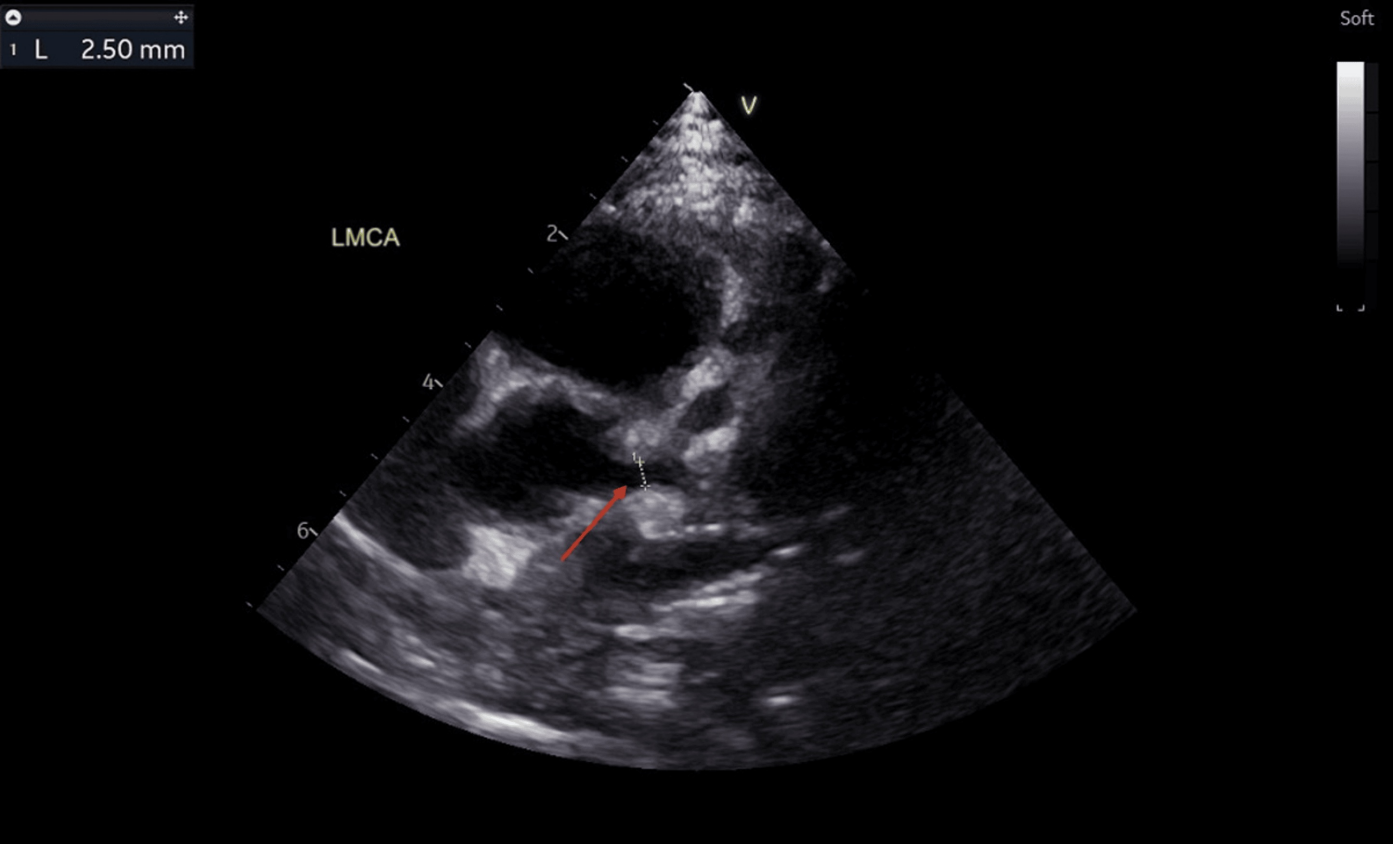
Echocardiogram showing reduction in the left main coronary artery dilatation. The red arrow points at the dilated left main coronary artery measuring 2.50 mm (Z score: +2.20).
